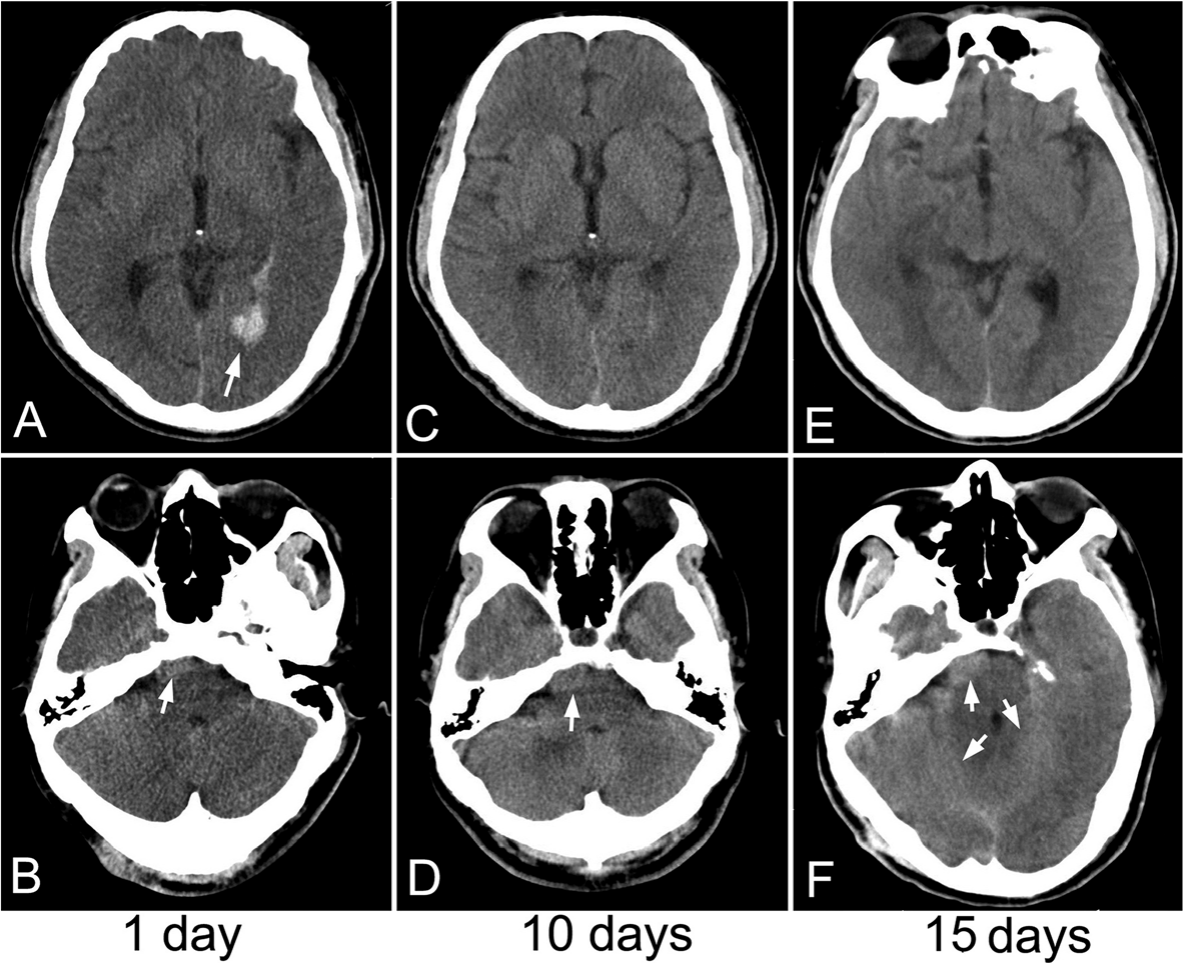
The dynamic change of cerebral CT after cerebral hemorrhage. At the first day, CT revealed hemorrhage in the left posterior horn of lateral ventricle (a, arrow), and a nodular mass with slightly high density at the right ventral surface of the pons (b, arrow). At the 10th day, CT scan showed hemorrhage almost disappeared (c), but the nodular mass still was unchanged (d, arrow). At the 15th day, CT showed hematoma was completely absorbed (e), but the nodular mass became more prominent accompanying abnormal density in the tentorium of cerebellum (f, arrow)
